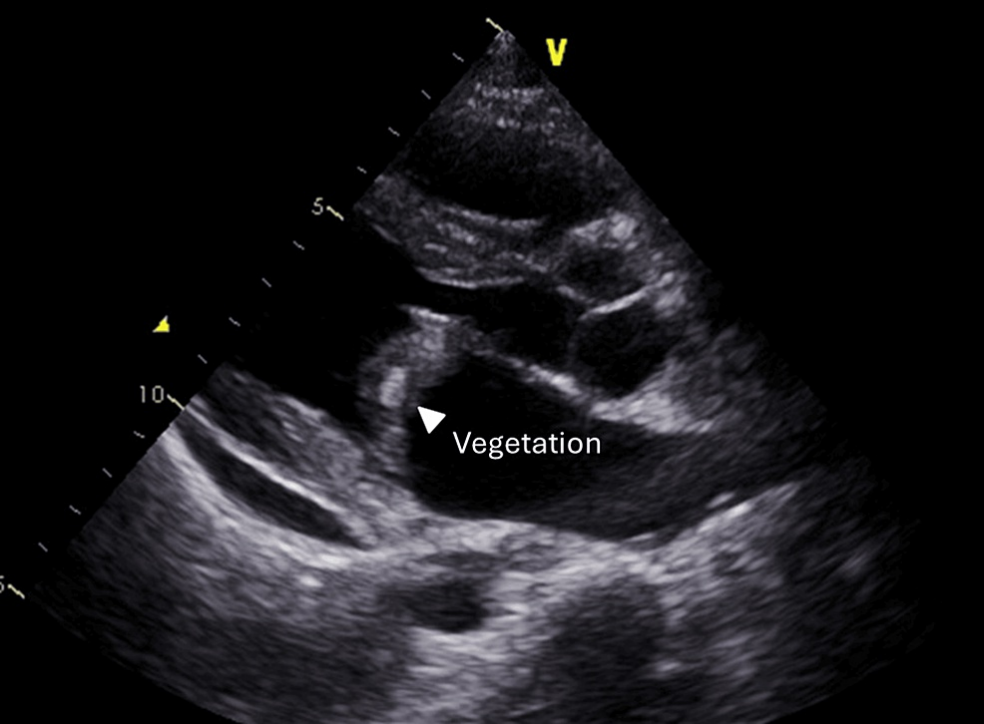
Transthoracic echocardiography showing vegetation on the mitral valve leaflet.
